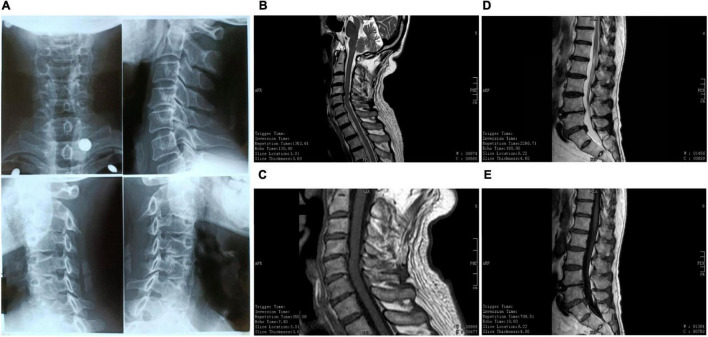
Spine. (A) Cervical X-ray examination in 2002. At that time, he was diagnosed with cervical degeneration and narrowing of the intervertebral foramen and intervertebral space. (B,C) Cervical magnetic resonance imaging (MRI) scan in 2020. (D,E) Lumbar MRI in 2020.
